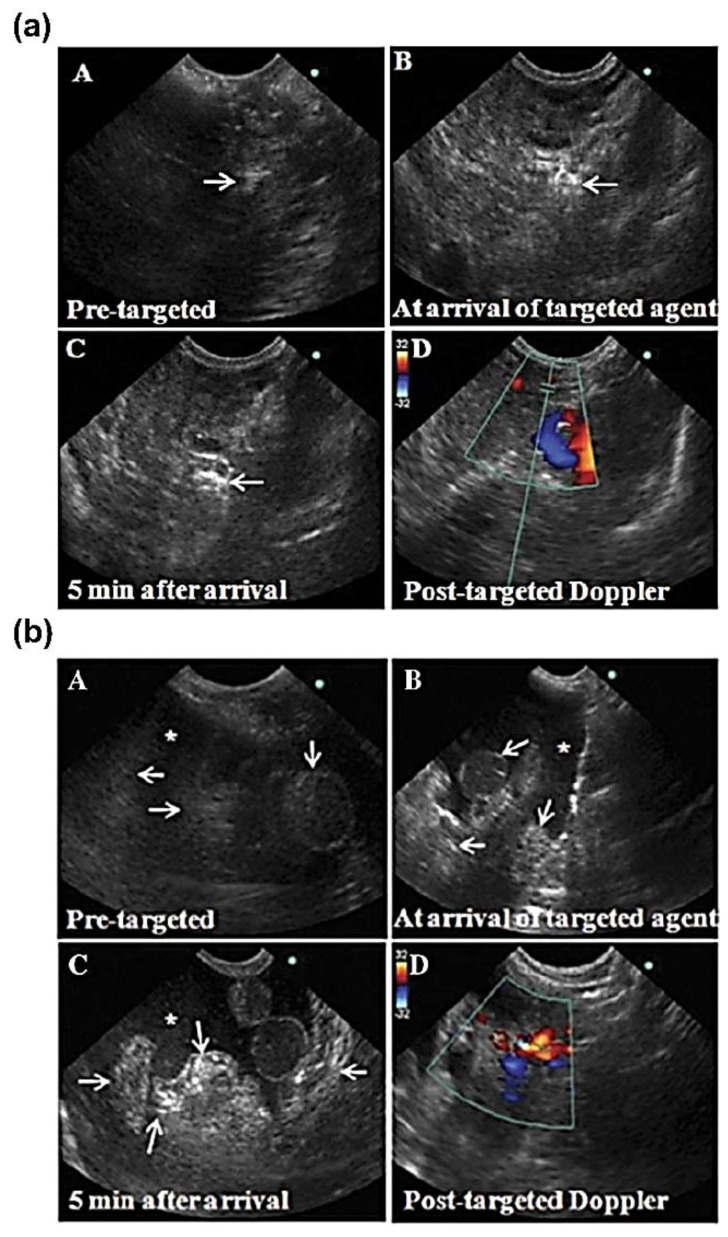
Targeted ultrasound microbubbles in spontaneous ovarian cancer egg-laying hen model by Barua et al. demonstrating the efficacy of relatively simple ultrasound molecular imaging agents in a native ovarian cancer model [114]. Commercially available microbubbles were functionalized with an affinity ligand, death receptor 6 (DR6), and subsequently intravenously administered and imaged at the across multiple time points, with peak intensity generated 5 min after the arrival of microbubbles to the site. The microbubbles significantly increased ultrasound imaging intensities measured via transvaginal ultrasound across early stage ovarian cancer (a), where the tumor was limited to the ovary, as well as late-stage disease (b). Imaging compared prior to the arrival of the microbubbles (A), post arrival (B,C) and a Doppler scan post-arrival of the microbubbles (D). Arrows indicate tumor seeding; * indicates profuse ascites.
